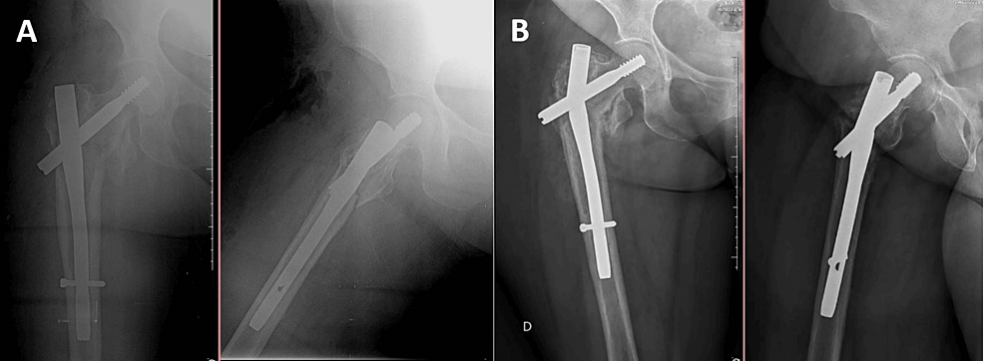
(A) Immediate postoperative radiograph and (B) after 12 weeks, showing implant breakage.
